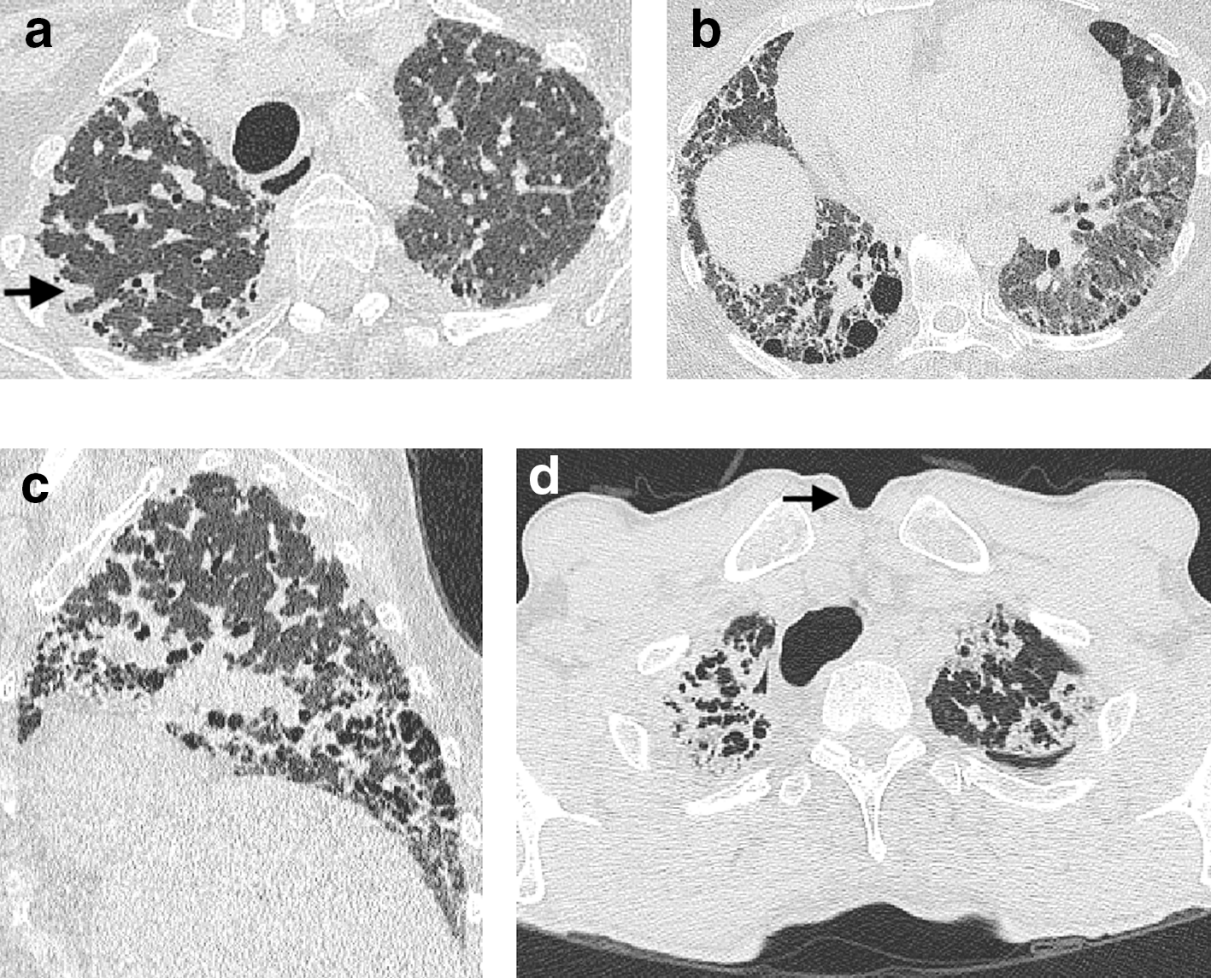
CT features of pleuroparenchymal fibroelastosis in a patient with idiopathic pulmonary fibrosis. Pleuroparenchymal fibroelastosis is characterized by pleurally based aggregations (arrow) of dense connective tissue in the lung apices (a). Honeycomb cysts in keeping with the underlying idiopathic pulmonary fibrosis diagnosis are evident at the lung bases (b + c). A suprasternal depression (arrow) and bronchiectasis in the upper lobes are also frequently associated with pleuroparenchymal fibroelastosis (d).
